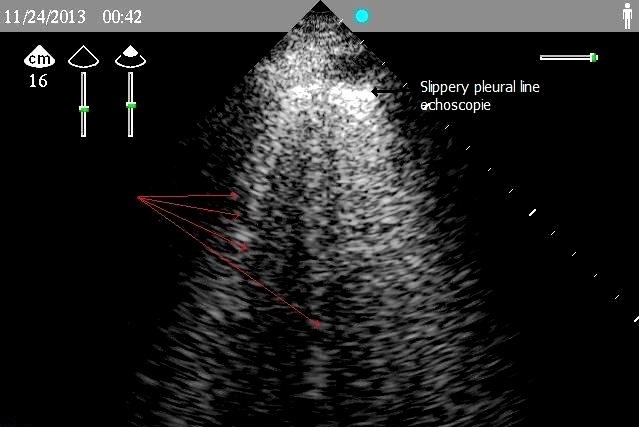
2D lung ultrasound showing the presence of several vertical lines B in favor of acute pulmonary edema (red arrows)
